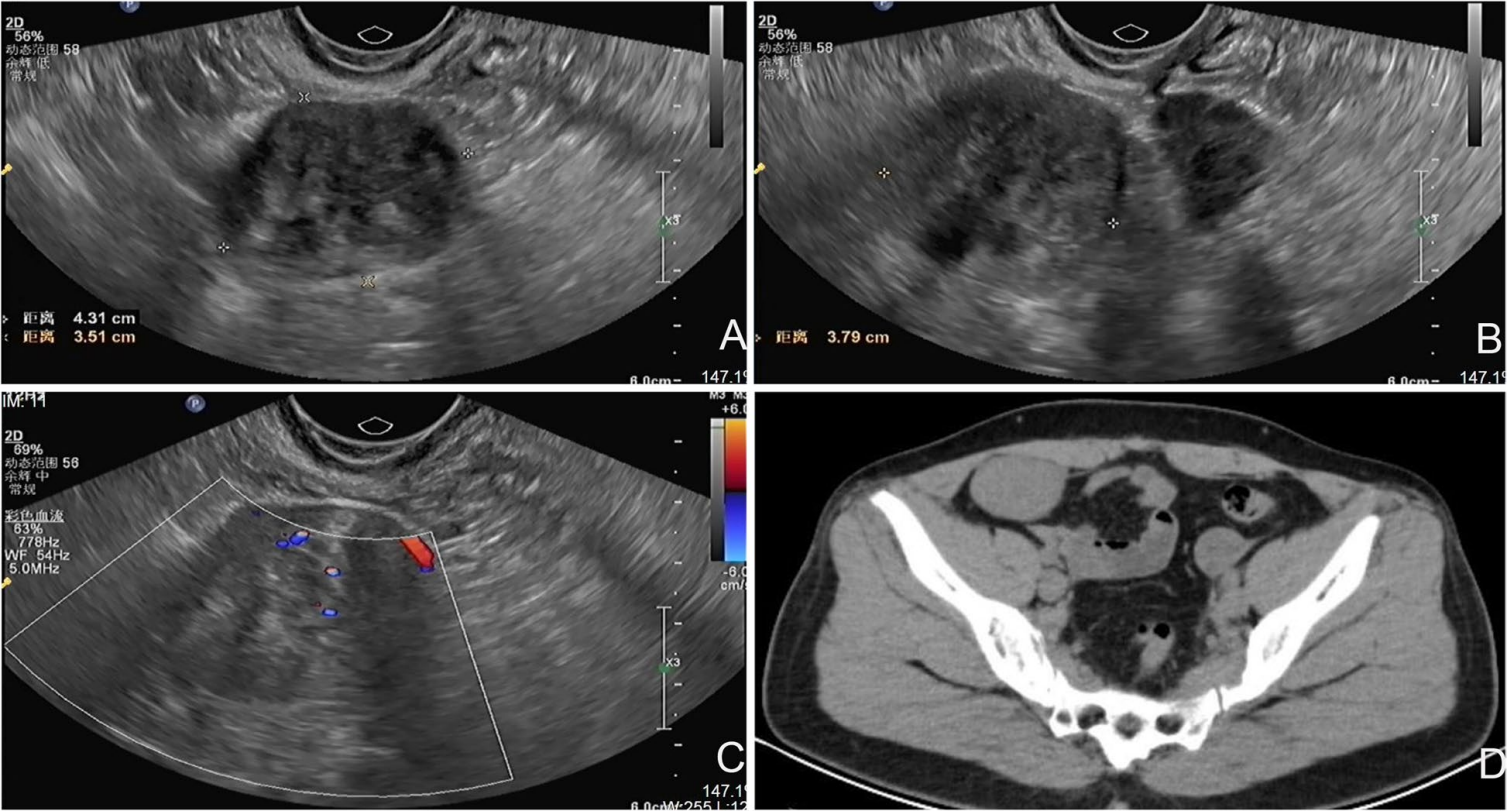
Imaging findings of the patient during the second hospitalization. A, B Pelvic ultrasound revealed a 4.3 × 3.8 × 3.5 cm mass in the right iliac fossa with a clear boundary and heterogeneous medium and low echo; C Color Doppler flow imaging revealed blood flow signals; D Abdominal CT revealed an 4.6 × 3.1 cm oval soft tissue density mass in the right iliac fossa. The CT value was approximately 53 Hounsfield units, the boundary was clear
